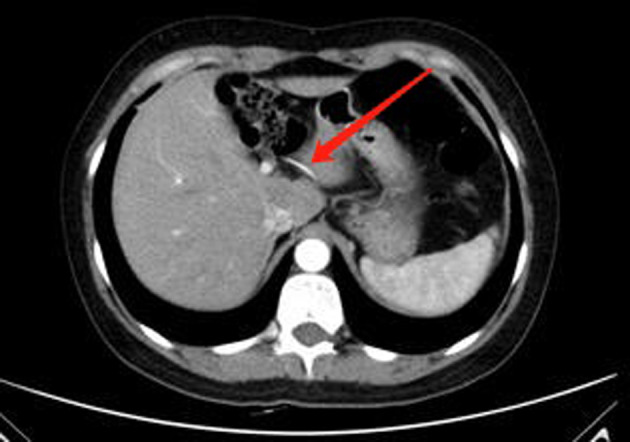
Computed tomography scan showing a hyperdense linear foreign body (red arrow) embedded in the liver.
